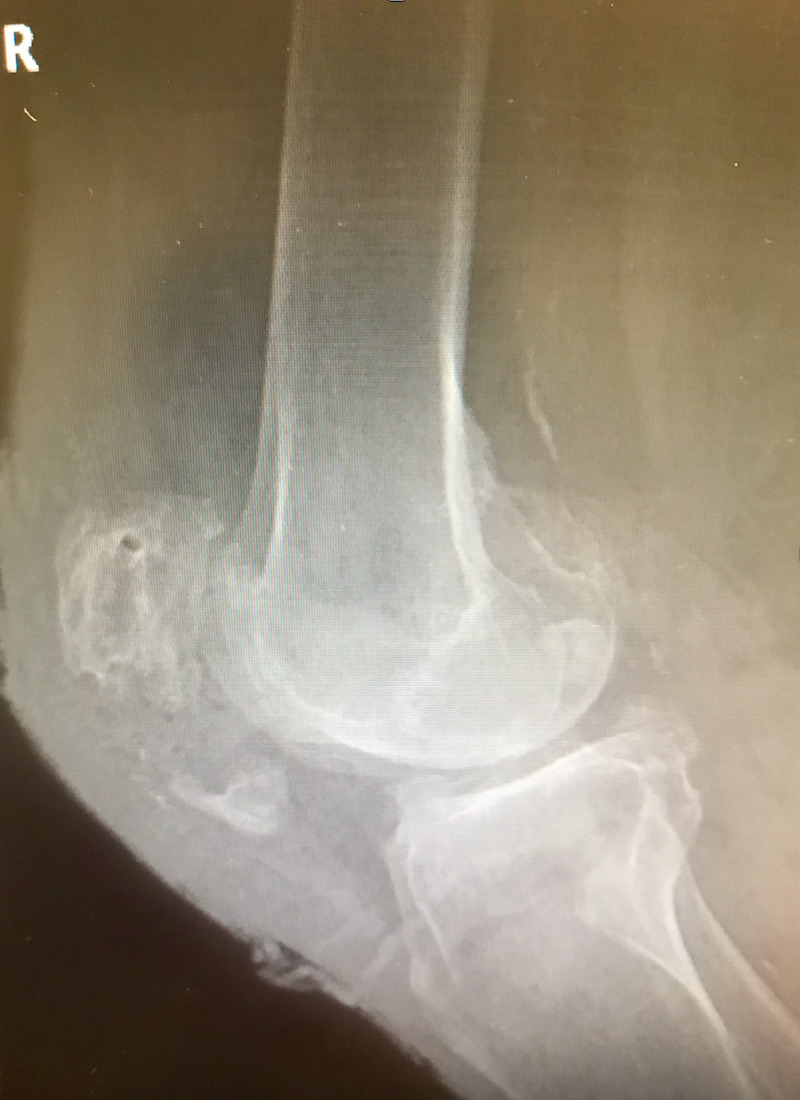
Lateral X-ray after fall Lateral X-ray view of the right knee following fall with wound dehiscence demonstrates new displaced horizontal patellar fracture of the inferior pole of the patella. Patella alta is noted.
